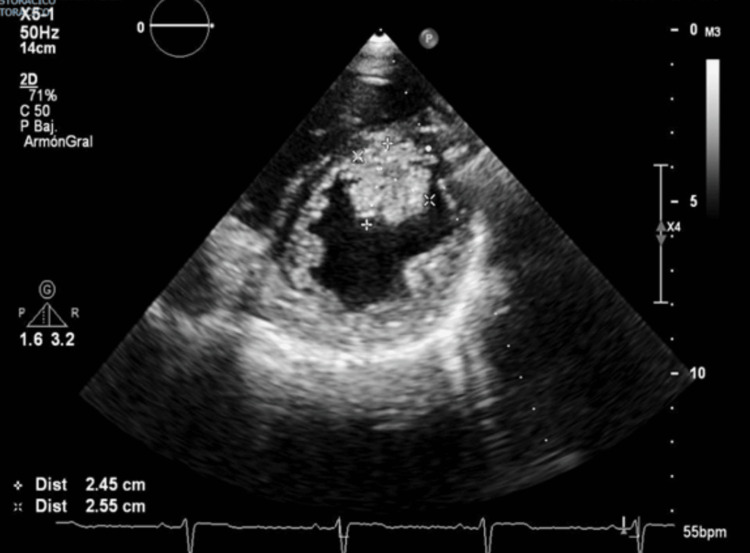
Parasternal short-axis view demonstrating a heterogeneous, intracavitary left ventricular mass with defined borders and measured dimensions of approximately 2.45 × 2.55 cm.
